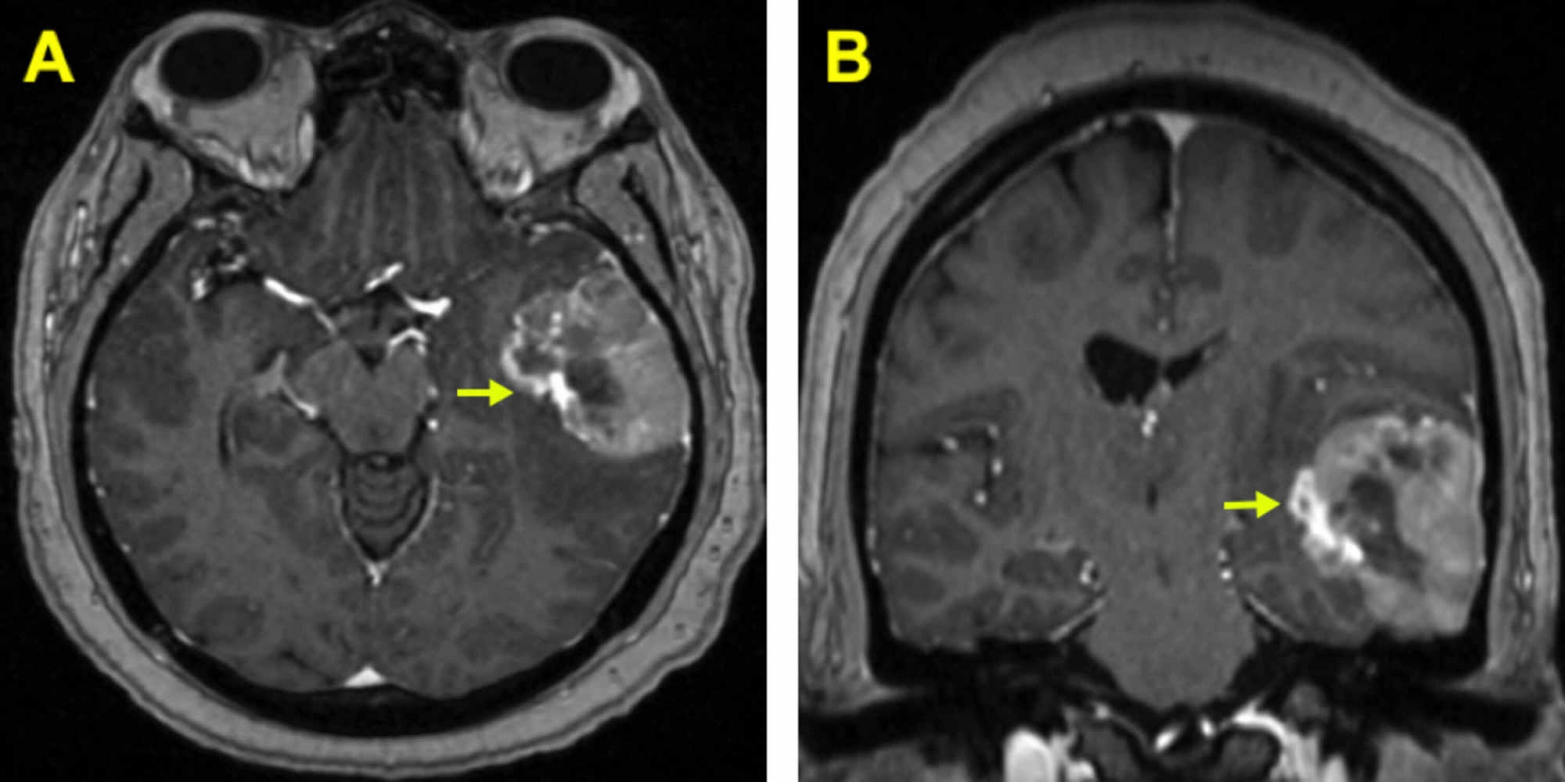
Preoperative MRI brain with contrast shows (A) left-sided temporal lesion (yellow arrow) (axial view), and (B) left-sided temporal lesion (yellow arrow) (coronal view).
